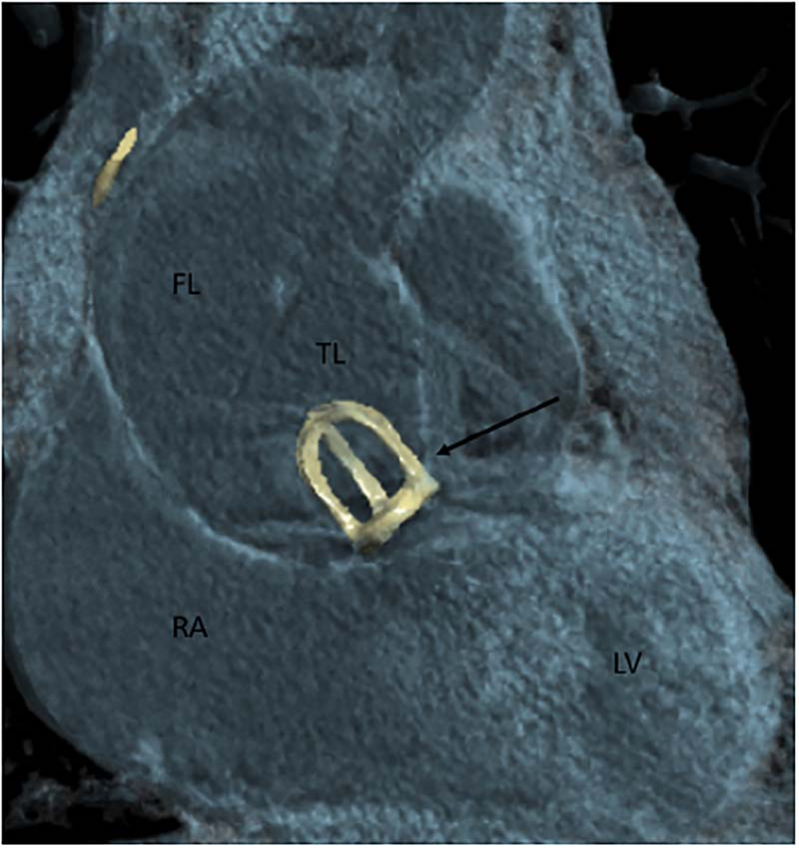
Contrast-enhanced CT aortography coronal plane in volume rendering technique reconstruction. Stanford A dissection distal to artificial caged-ball (thin arrow) mechanical valve. FL, false lumen; TL, true lumen; RA, right atrium; LV, left ventricle.
